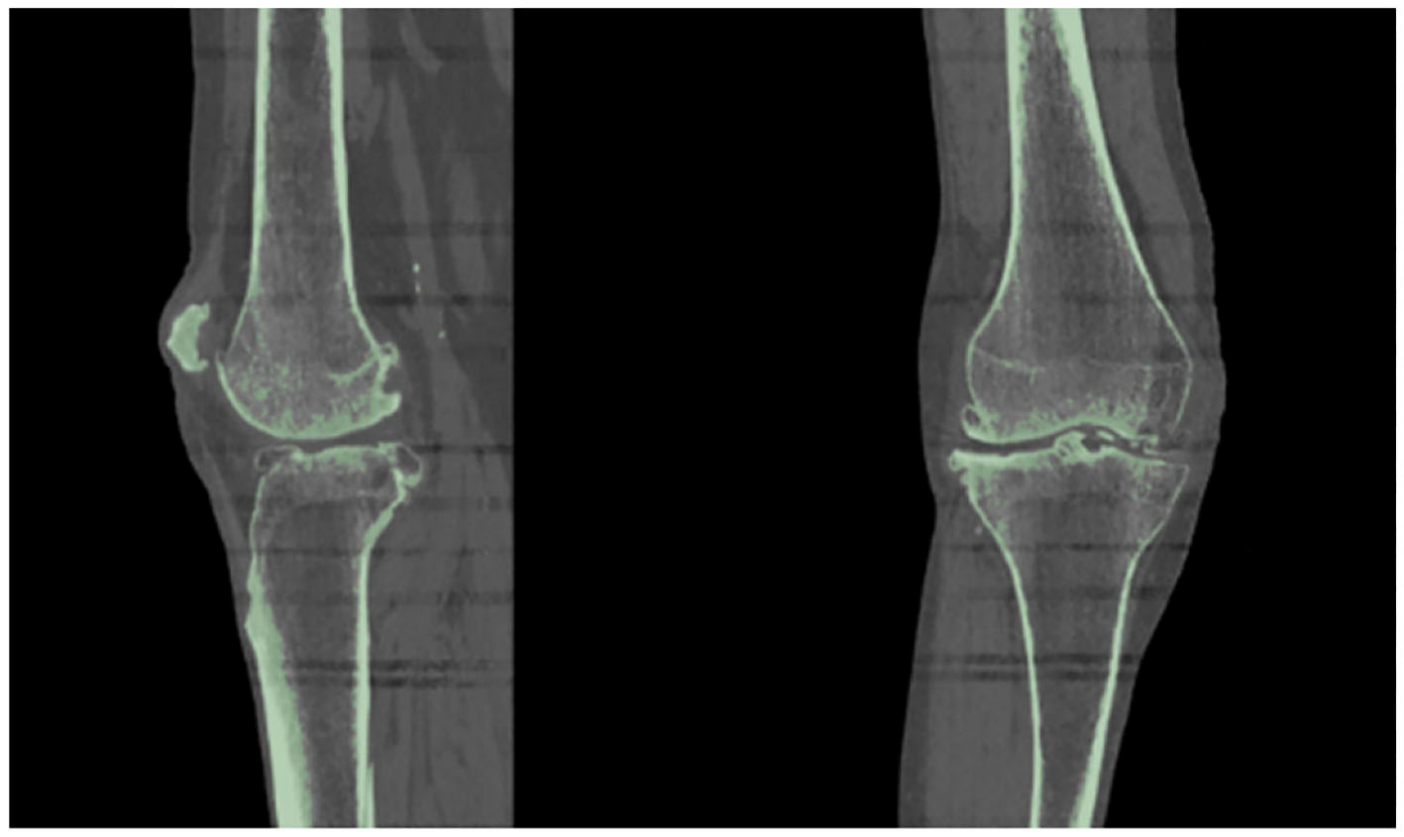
Results obtained using 3D Slicer's “Threshold” algorithm. Since the bones have the same intensity in the CT image, the software corresponds one label (indicated with green color) to all the pixels representing the patient's knee bones.
